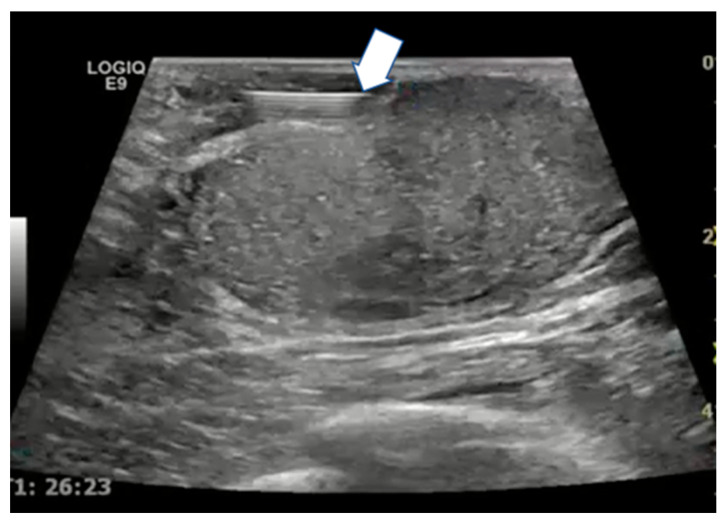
Ultrasound scan with linear probe showing the tip of the needle used for hydrodissection (arrow) to inject saline between the tumor and the skin or ductal structures.
